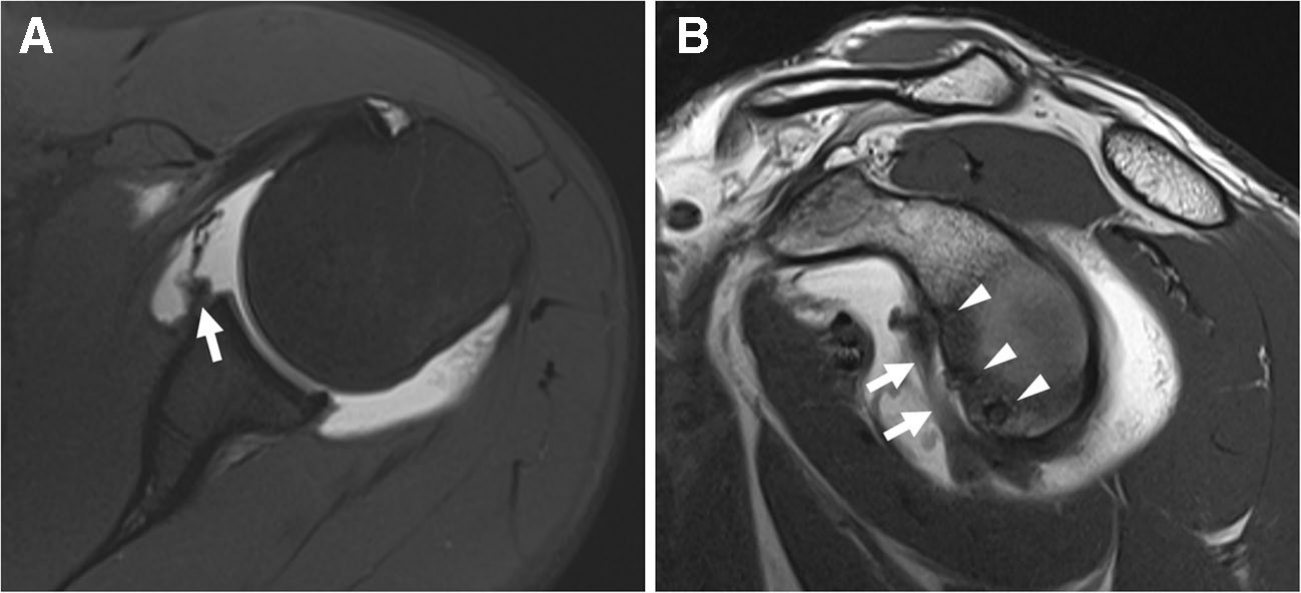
Recurrent anterior labral tear. Twenty-six-year-old man with anterior glenohumeral dislocation 1 year following arthroscopic Bankart repair. Axial T1-weighted fat-suppressed (A) and sagittal T1-weighted (B) 1.5 T MR arthrogram images demonstrate medialized and scarred anteroinferior labroligamentous tissue (arrows), consistent with a chronic anterior labroligamentous periosteal sleeve avulsion (ALPSA). Note the anterior glenoid anchor tracks (arrowheads)
